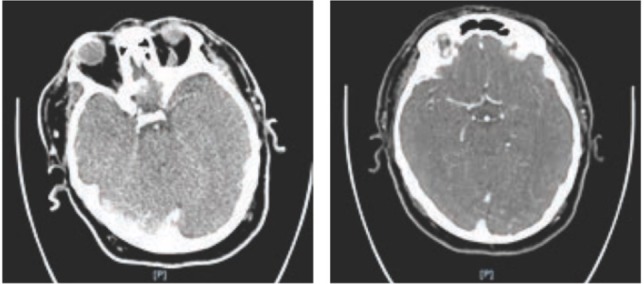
Native CT showing hyperdense left MCA, and CT angiography showing occlusion of the M2 branch of the left MCA.
